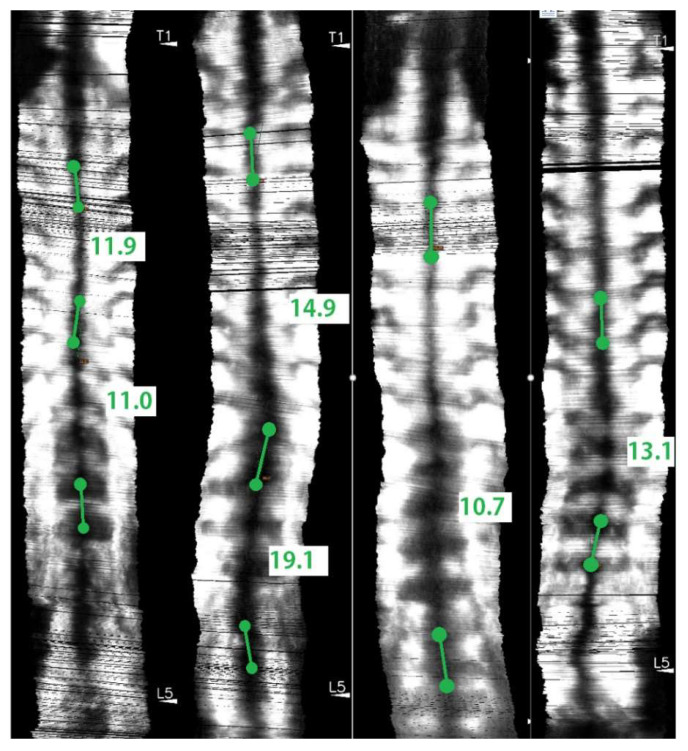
Coronal ultrasound images of subjects with various scoliosis severities using Scolioscan Air with SPA measurement.
